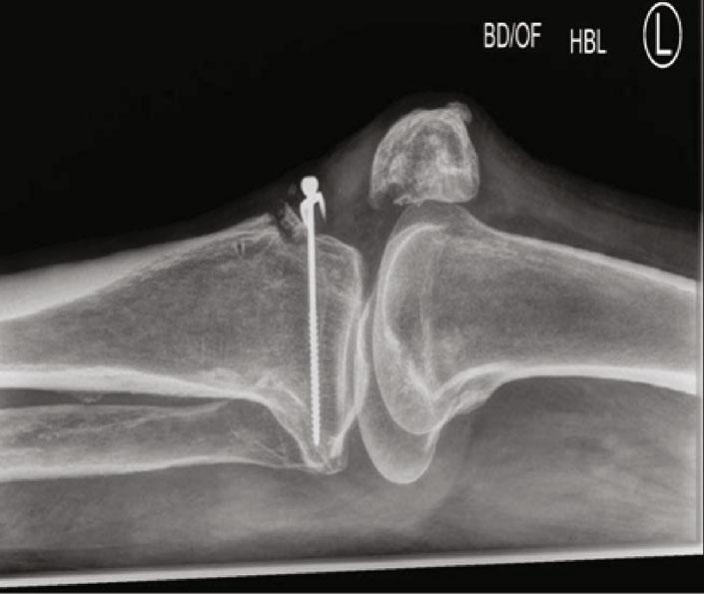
Lateral radiographs of the knee after the second injury with migration of the washer and failure of fixation.
